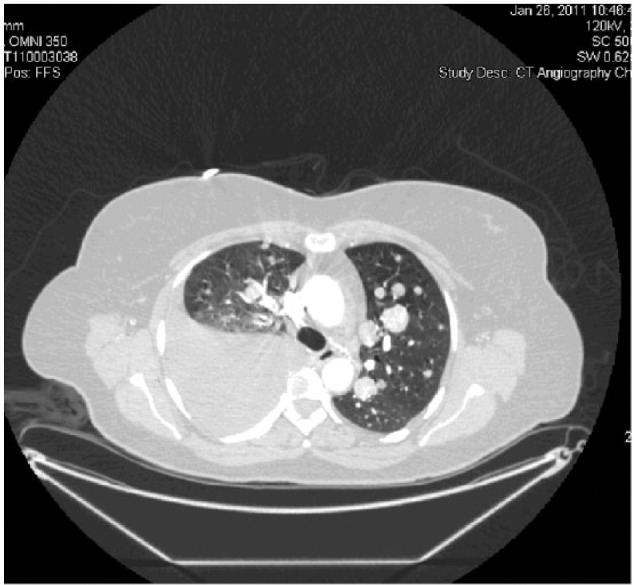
Computed tomography scan of the chest, abdomen, and pelvis revealed too numerous to count bilateral pulmonary nodules with a large pleural effusion, and near complete collapse of the right middle and right lower lobes.
